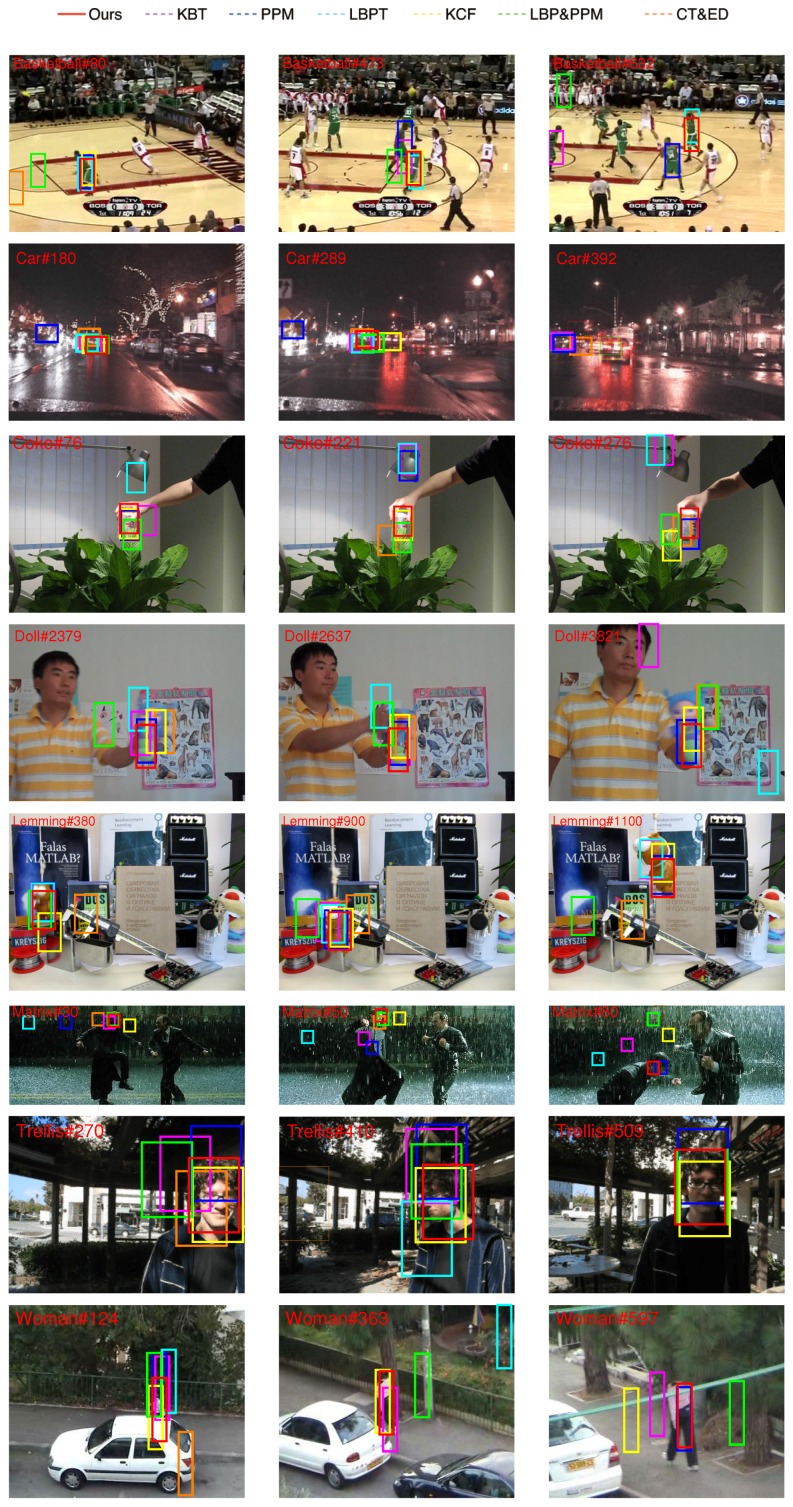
Experiment results of our proposed algorithm, KBT [10], PPM [15], LBPT [20], KCF [9], LBP&PPM and CT&ED on eight challenging sequences (from top to bottom are Basketball, Car, Coke, Doll, Lemming, Matrix, Trellis, Woman, respectively).
